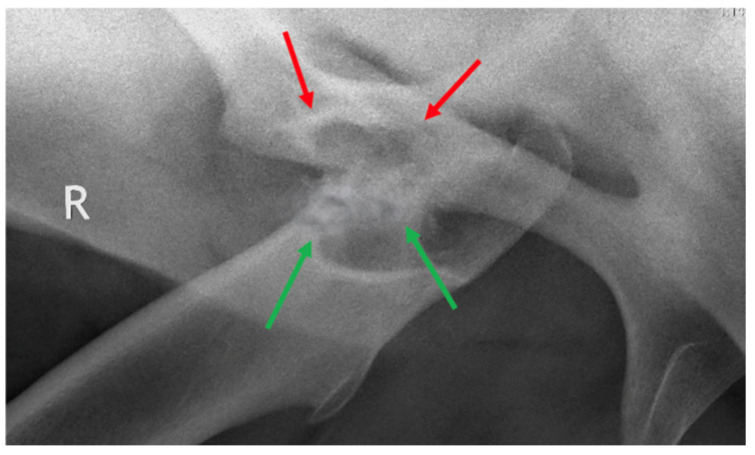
Standing medial 30° cranial 20° dorsal-lateral caudal oblique radiographic view of the right coxofemoral joint, 5 years post-op. A pseudoarthrosis is evident. The acetabulum (red arrows) appears more shallow and smaller than pre-operatively. Large and irregular new bone formation at the femoral ostectomy site (green arrows) is clearly visible. The cranial is to the left.
